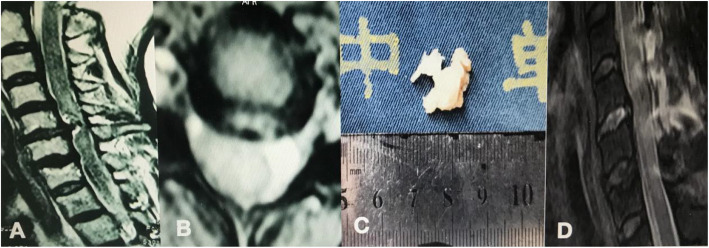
A 66-year-old female patient presented with Brown-Sequard syndrome of the left limbs and trunk for 3 days and was diagnosed with rp-CSM. a, b MRI showed spinal compression and T2-hyperintensity at the C5/6 disk level with edema at the C4–5 and C5–6 levels, probably due to spinal cord ischemia. c The patient underwent laminectomy and fusion, and the large disk was removed. d Postoperative MRI showed that decompression was satisfactory and neurological symptoms improved significantly
